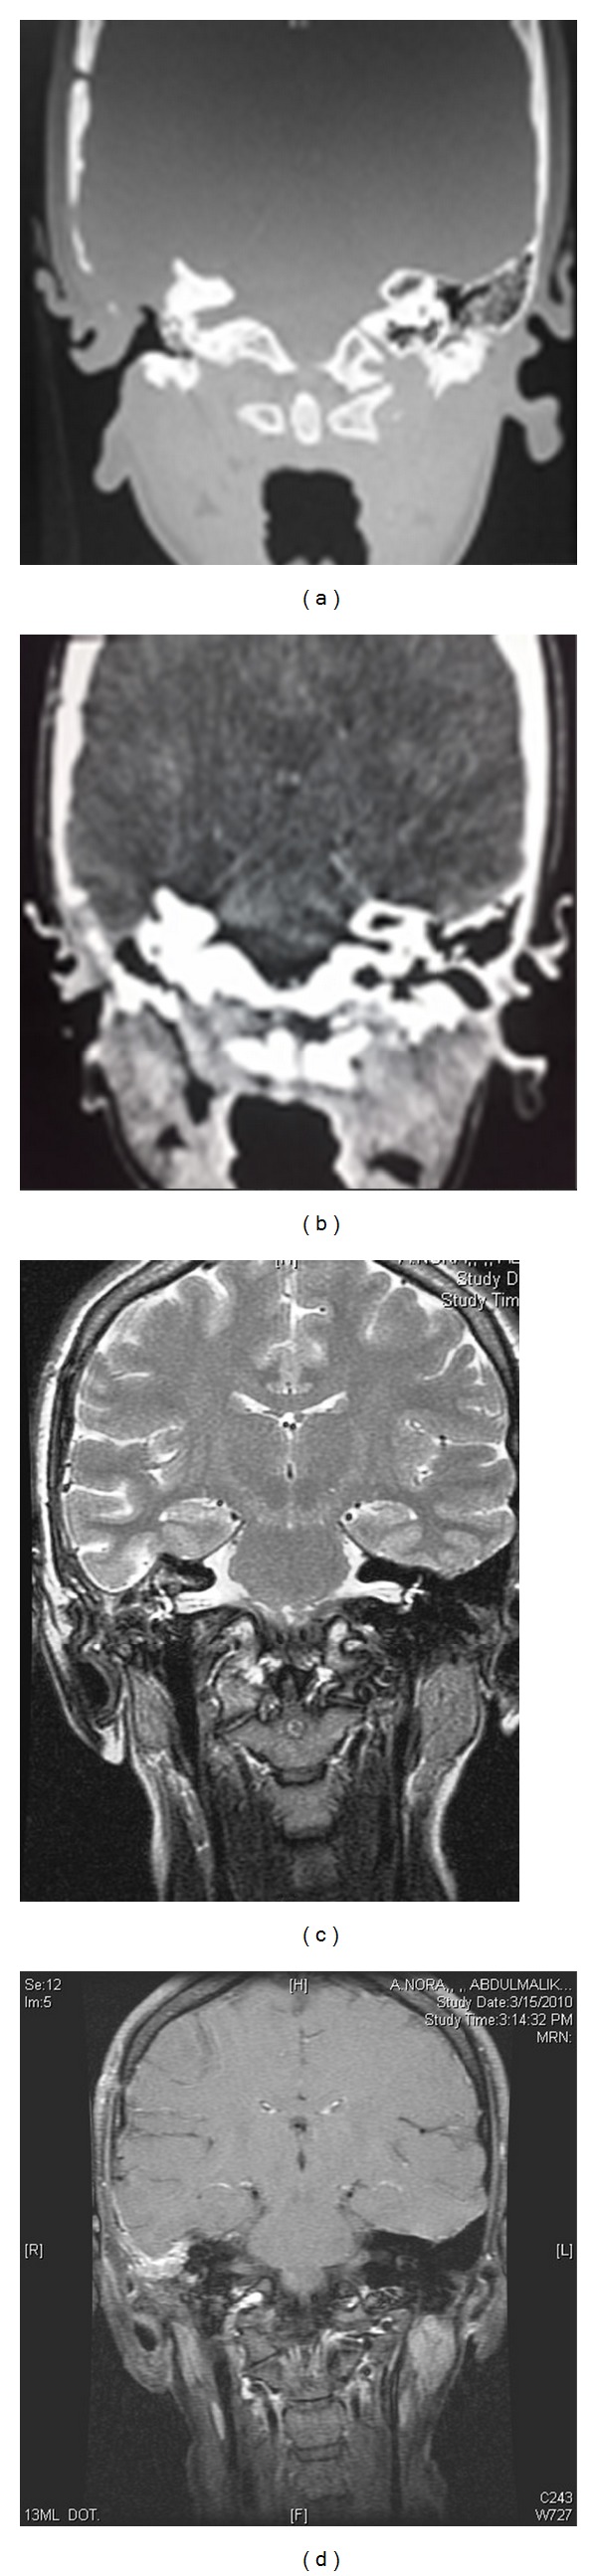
Three months after surgery, the CT (a, b) and MRI (c, d) of the temporal bone and brain revealed complete resection of the dermoid cyst and return of the right temporal lobe of brain to its normal position.
